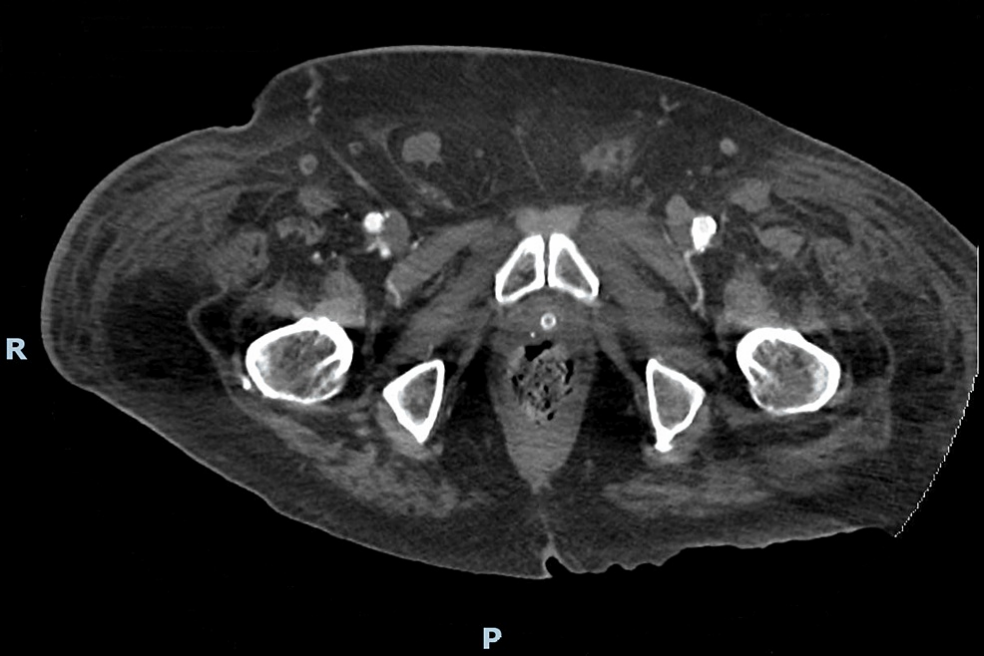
Pelvic computed tomography showing edematous infiltration of the subcutaneous tissues, more exuberant on the right, consistent with cellulitis of the lower limb. R: right; P: posterior
